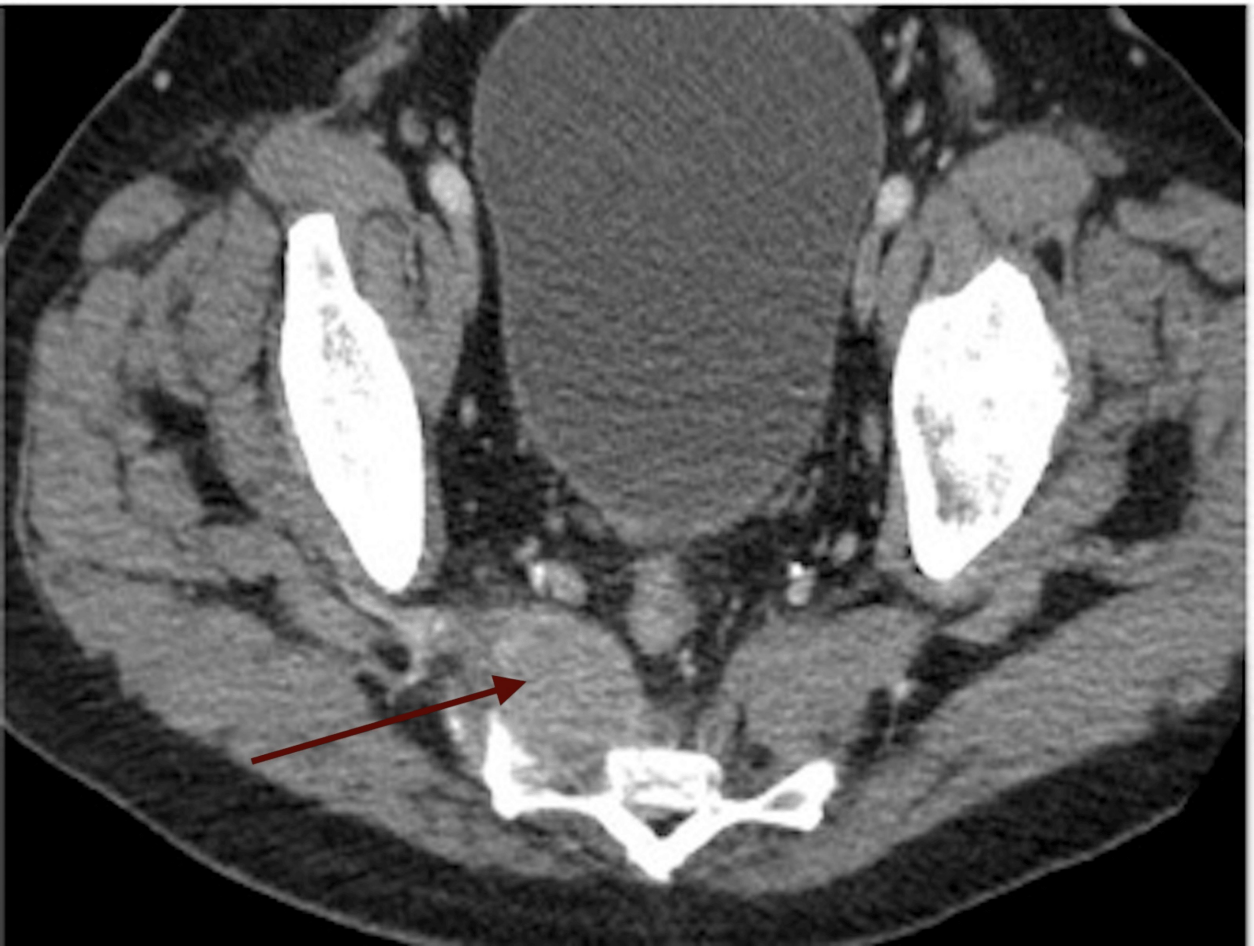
CT image of the lesion at the level of S2-S3 sacral body with malignant extension into the piriformis muscle The red arrow indicates a metastasized tumor within the piriformis muscle. CT: Computed tomography
